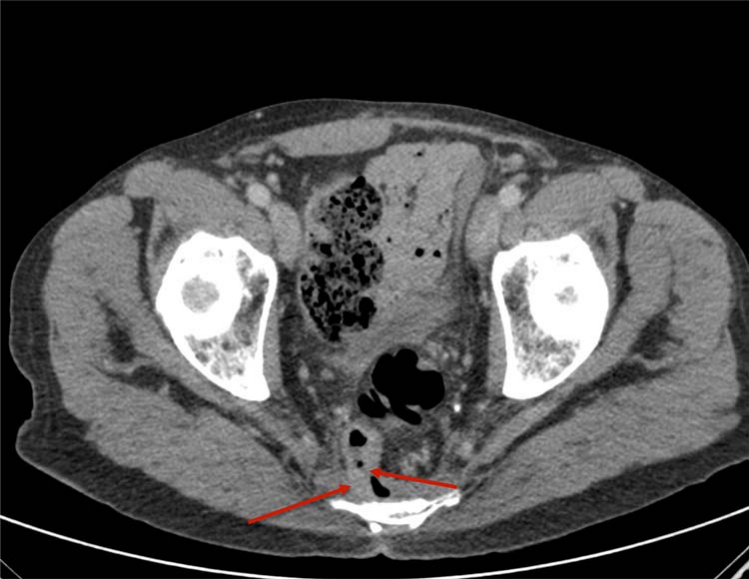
Axial section of contrast-enhanced CT (CECT) shows the communication of rectum to presacral collection at anastomotic site.
